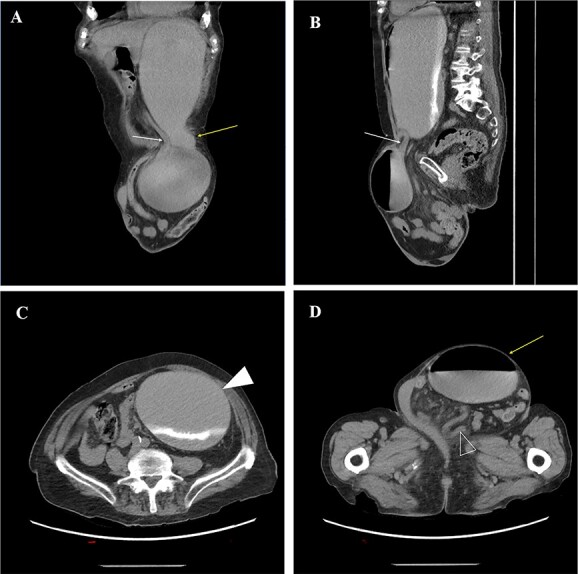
(A) oblique coronal reconstructions from a non-contrast CT demonstrates the gastric body (yellow arrow) entering the left inguinal hernia, and the obstructed gastric antrum (white arrow) exiting the hernia; (B) oblique sagittal reconstruction from a CT demonstrates the obstructed gastric antrum (white arrow); (C) axial non-contrast CT through the abdomen demonstrates a dilated gastric body and fundus (white arrowhead) with layering contrast fluid and ingested material; (D) axial non-contrast CT image through the pelvis demonstrates a large left inguinal hernia containing the obstructed gastric body (yellow arrow) and multiple loops of decompressed small bowel (open arrowhead).
